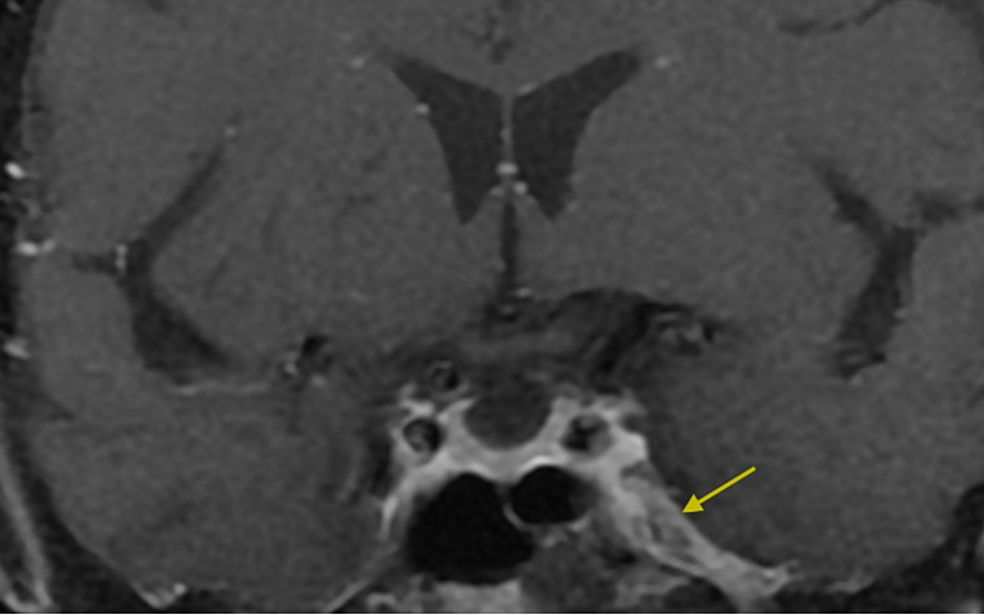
Gadolinium-enhanced coronal T1-weighted 1.5-Tesla MRI images of the brain showing mandibular nerve enhancement in the left foramen ovale (yellow arrow).
